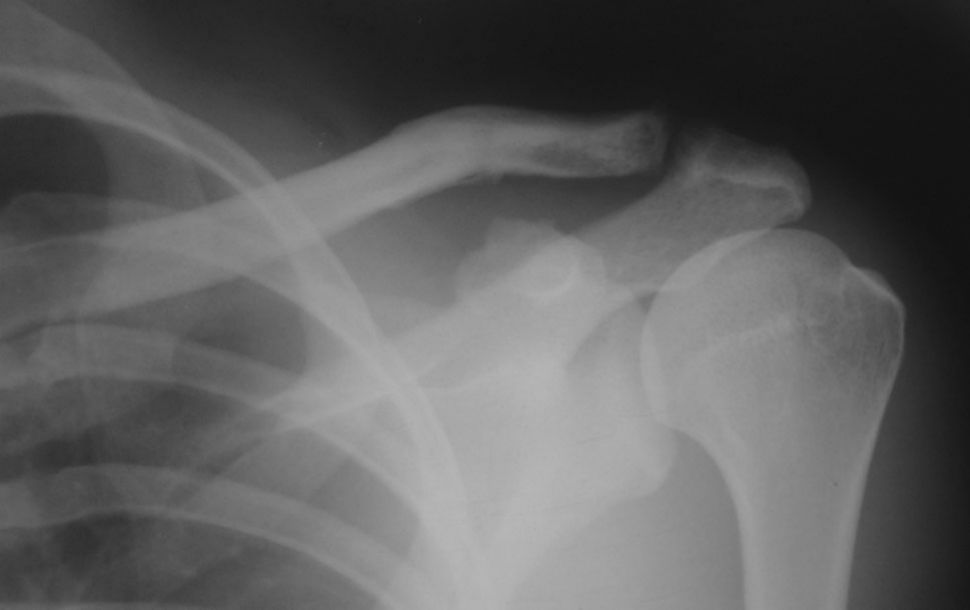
Stress radiograph at 2 years post hook plate fixation: Congruent acromioclavicular articulation and normal coracoclavicular distance with mild sclerosis
